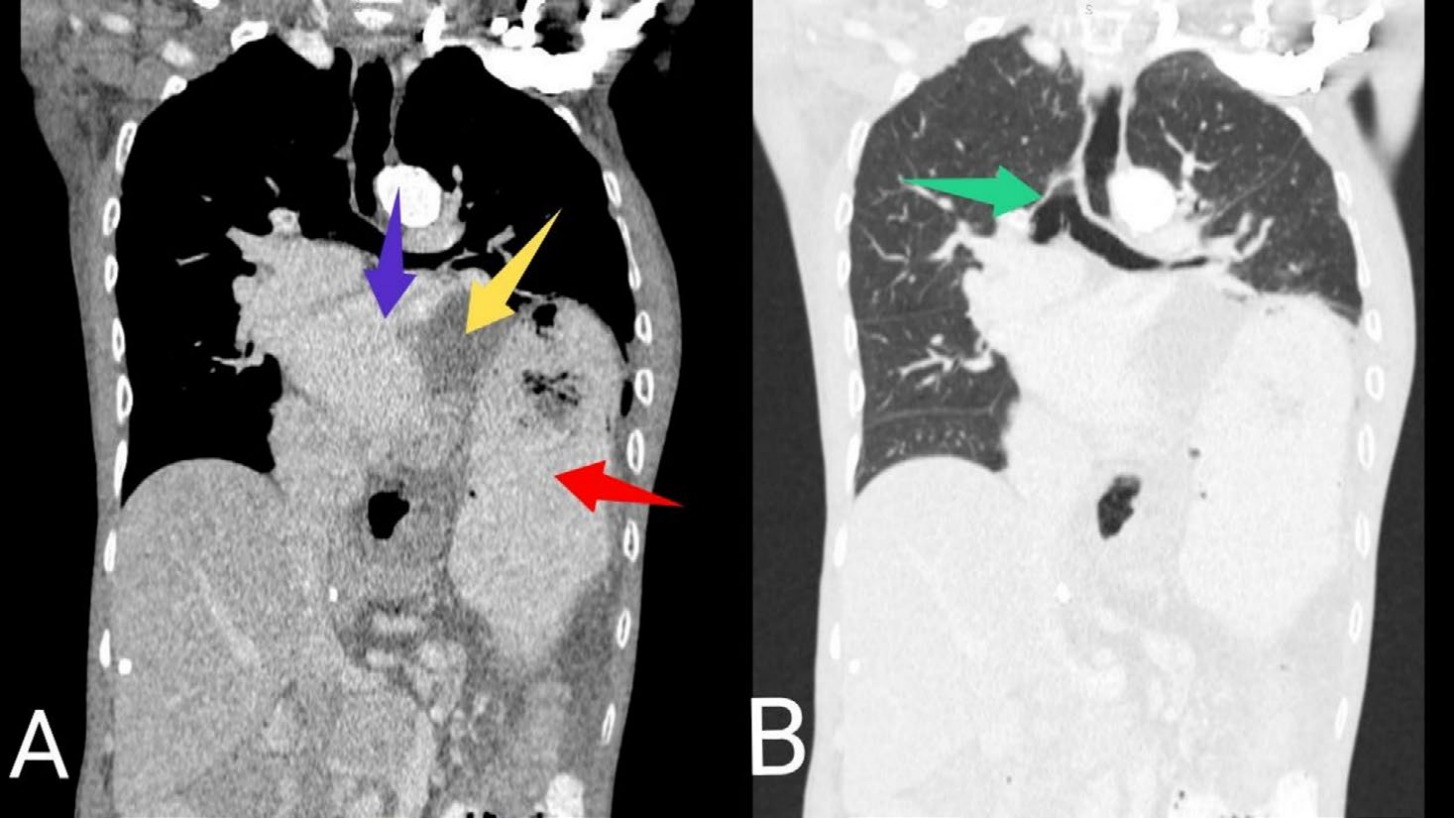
Contrast‐enhanced coronal chest CT scan images in mediastinal (A) and lung (B) windows showing the stomach (red arrow in A) and mesenteric fat (yellow arrow in A) directly compressing the heart (blue arrow in A) with associated minimal rightward mediastinal shift (green arrow in B).
